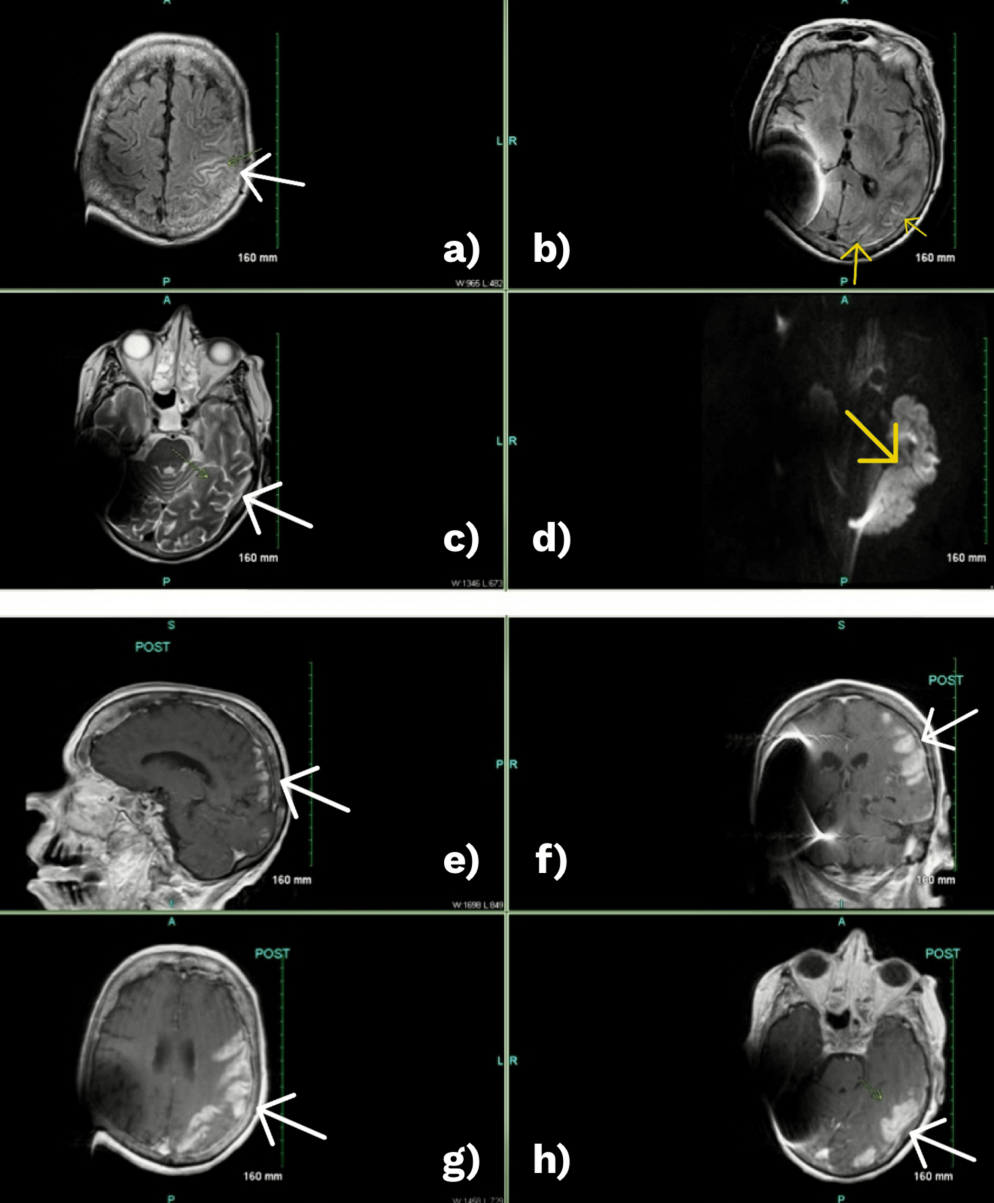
Follow-up MRI brain on hospital day 12 MRI of the brain demonstrating evolution of findings consistent with acute hemorrhagic leukoencephalitis (AHLE). a: axial fluid attenuated inversion recovery (FLAIR) image showing cortical hyperintensity and sulcal effacement in the left parietal region (white arrow); b: axial T1-weighted image revealing petechial hemorrhages and mass effect with early midline shift (yellow arrows); c: axial T2-weighted image highlighting vasogenic edema in the left cerebellar hemisphere (white arrow); d: diffusion-weighted image (DWI) showing restricted diffusion in the left hemisphere (yellow arrow); e–g: post-contrast T1-weighted sagittal, coronal, and axial images demonstrating cortical and leptomeningeal enhancement with associated mass effect and hemorrhages (white arrows); h: post-contrast axial image at the level of the brainstem showing additional abnormal enhancement (white arrow).
